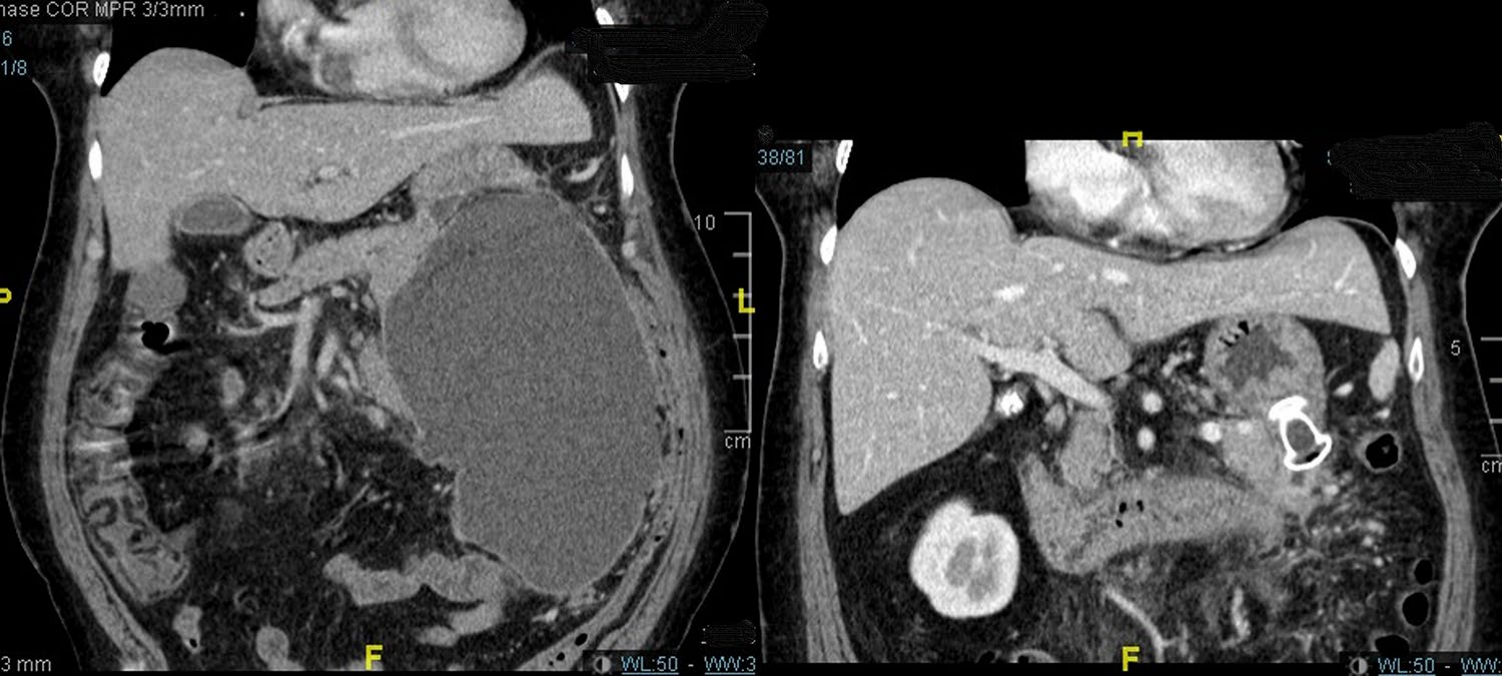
Computed tomography imaging of pancreatic walled-off necrosis before (left) and after (right) endoscopic ultrasound-guided lumen-apposing metal stent placement and direct endoscopic necrosectomy.
